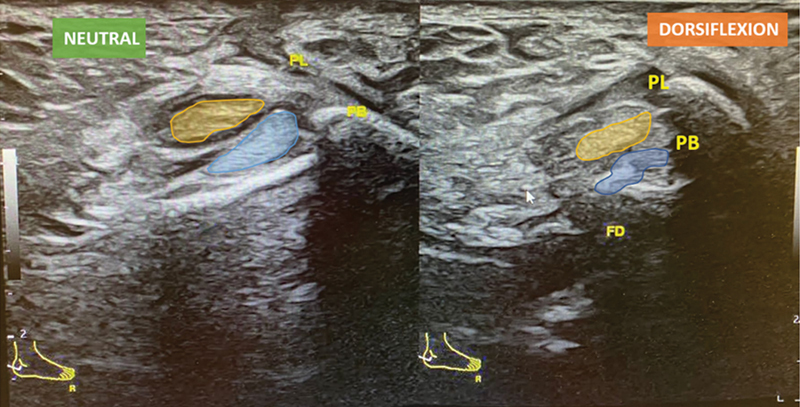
Postoperative dynamic ultrasound: normal retromalleolar positioning of the peroneal tendons both at rest (left) and in dorsiflexion (right). Abbreviations: PL, peroneal longus tendon; PB, peroneal brevis tendon.
